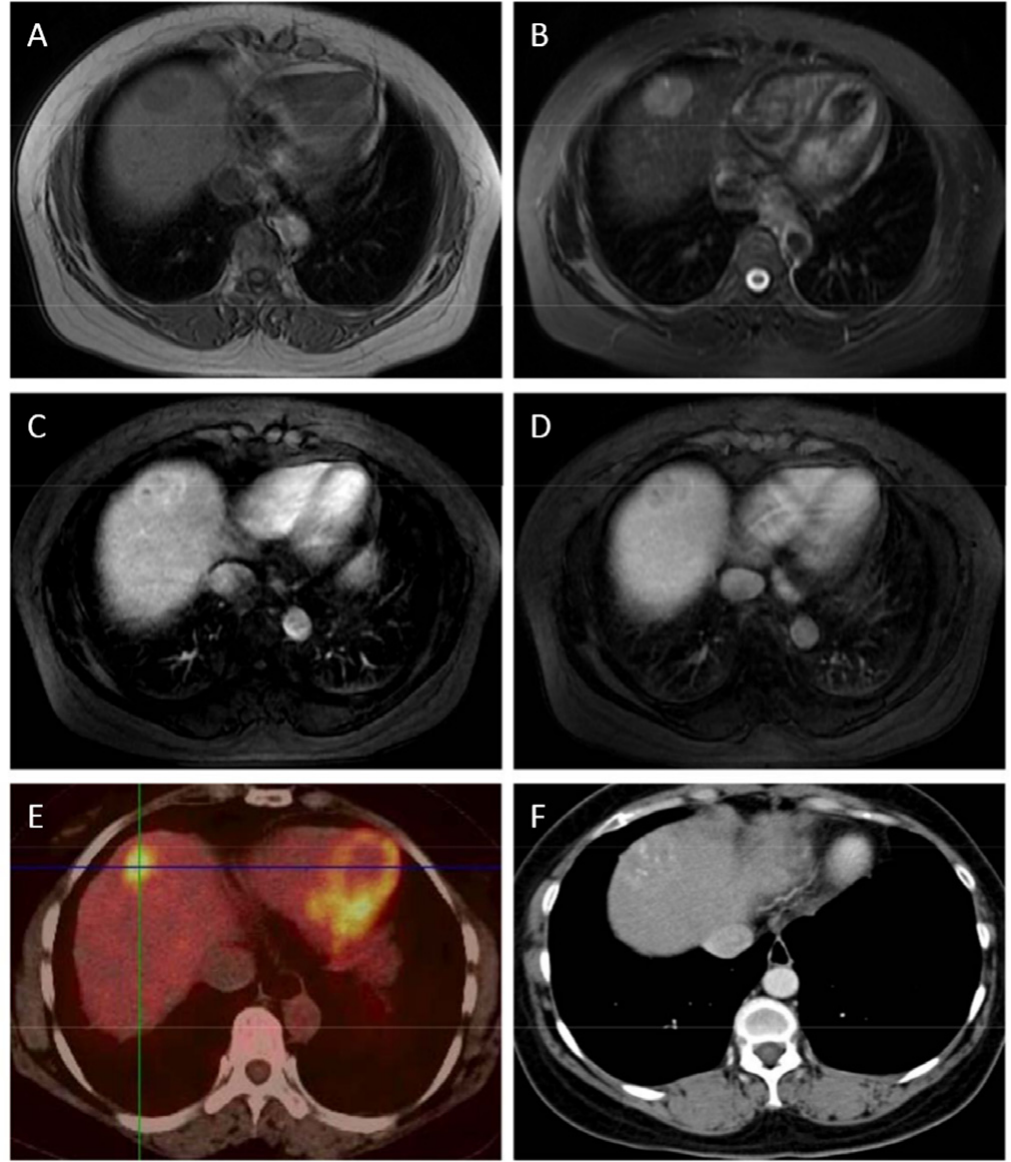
A: A 2.9*2.2cm sized round lesion is seen in the segment 8 of the liver which shows low signal intensity on T1-weighted MR image. B: A 2.9*2.2cm sized round lesion is seen in the segment 8 of the liver which shows slightly high signal intensity on T2-weighted MR image. C: Late arterial phase of dynamic contrast enhanced scan shows inhomogeneous enhancement in the lesion with obvious enhancement on the border. D: Portal venous phase of dynamic contrast enhanced scan shows the overall enhancement has not washed out. E: PET-CT reviews: increasing abnormal uptake with SUV max 6.8. F: CT scan shows punctate distribution lipiodol deposition inside the lesion after TACE.
